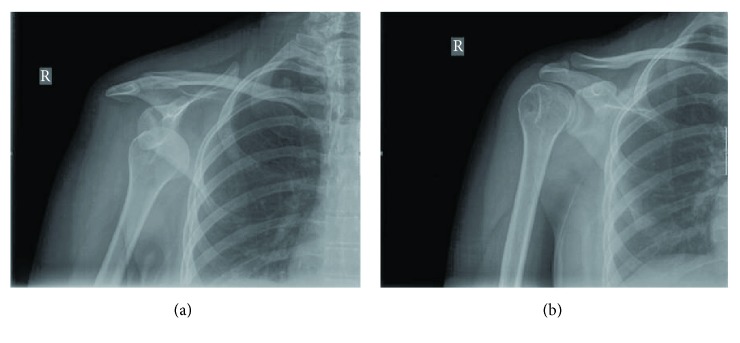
Radiographs of the shoulder demonstrate anterior shoulder dislocation (a) and her postreduction (b).
